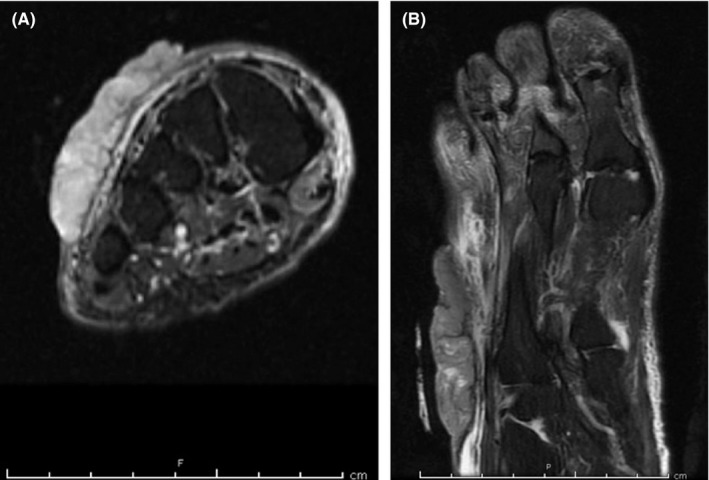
Preoperative magnetic resonance imaging of the lesion in (A) T2‐weighted and (B) short‐tau inversion recovery sequencing
